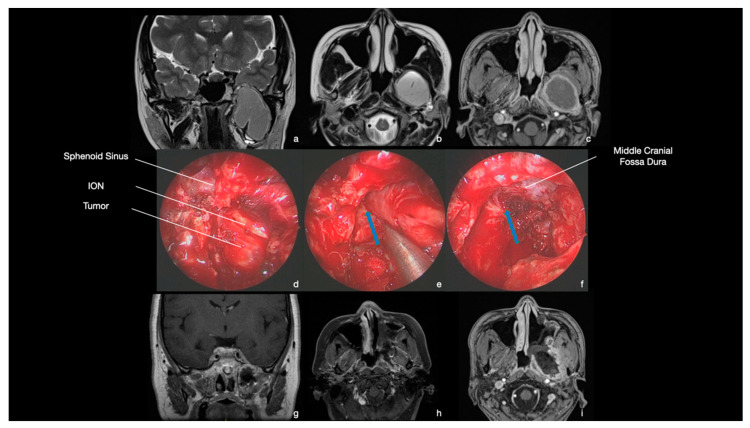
MRI and intraoperative endoscopic images (0° scope). (a–c) MRI. Coronal and axial T2 w-sequence and T1 w-sequence with contrast agent. A large tumor was observed in the left PPF and ITF. (d) Intra-operative image. After medial maxillectomy, the posterior wall of the maxillary sinus is removed, and the tumor is exposed. The mass was of firm consistency and diffusely bleeding. (e) Intra-operative image. Tumor was progressively resected, and the mandibular nerve was identified and anatomically preserved until the foramen ovale (blue arrow). The mass was cleaved from middle cranial fossa dura, without any CSF leak. (f) Intra-operative image. At the end of the surgery, the tumor was radically removed, with preservation of anatomical structures. (g–i) MRI. Coronal and axial T1 w-sequence with contrast agent. Complete tumor removal was demonstrated.
